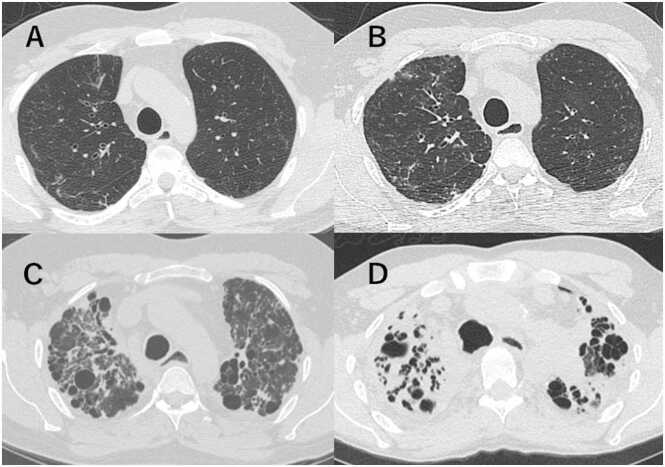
A 34-year-old male patient with an upper-predominant case underwent serial chest CT scans over a 10-year period. (A) Initial CT at CLAD diagnosis showed ground-glass opacities (GGOs), small linear and reticular opacities predominantly in the right upper lobe. (B) One year after diagnosis, a progressive increase in the right lung opacities was demonstrated, with new GGOs and small nodules appearing in the left upper lobe. (C) Five years after diagnosis, marked volume loss was revealed in both lungs, with the development of consolidation accompanied by bronchiectasis. (D) Ten years after diagnosis, dense consolidation was observed with bronchiectasis and cystic changes. Notable volume loss is evident in both upper lobes. The patient died 11.5 years after CLAD diagnosis. CLAD, chronic lung allograft dysfunction; CT, computed tomography.
